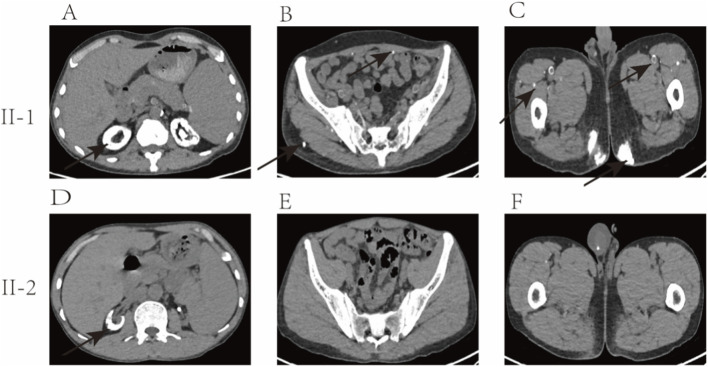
Radiological findings in the two affected siblings. (A–C) Axial computed tomography images of the proband (II-1), showing bilateral renal atrophy with diffuse calcification (A), pelvic soft-tissue calcification (B), and gluteal soft-tissue calcification (C). (D–F) Corresponding computed tomography images of the brother (II-2), showing bilateral renal atrophy with diffuse calcification (D), without apparent pelvic soft-tissue calcification (E) or gluteal soft-tissue calcification (F). Arrows indicate the relevant abnormalities.
